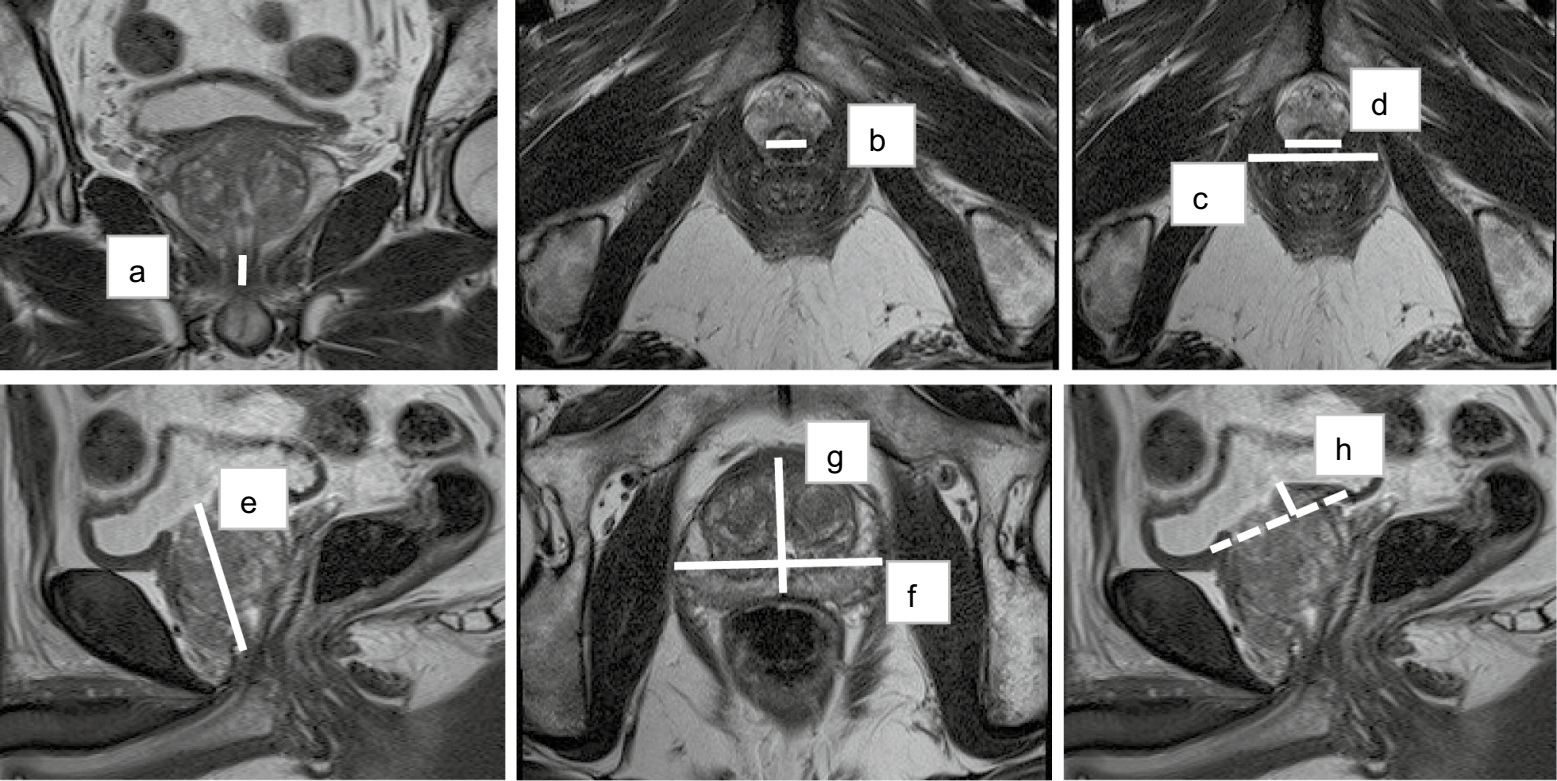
MRI measurements. *a* is the MUL. MUL was measured as the distance of the apex of the prostate to the base of the bulbous urethra. *b* is the MUW. MUW was defined as the maximum diameter of the membranous urethra. LT was calculated as (*c* − *d*)/2. *c* stands for the outer levator distance and *d* stands for the inner levator distance. PV was calculated as *e* × *f* × *g* × π/6/1000. *e* is the maximum height of the prostate, *f* is the maximal prostate width and *g* is the maximal prostate length. Intravesical prostatic protrusion [*h* (mm)], which is measured from the tip of the protruding prostate to the base of the bladder (mid-sagittal T2-weighted image)
